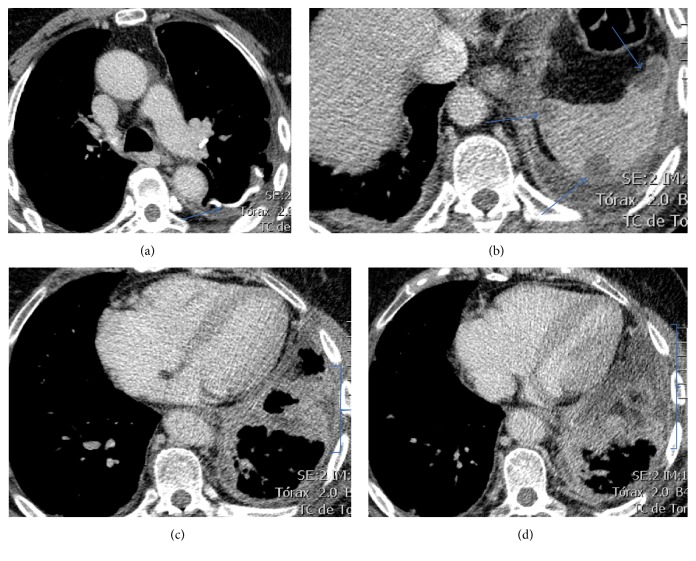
Chest CT. Multislice chest axial CT-images on mediastinal window show (a) signs of previous atypical wedge resection in the left lower lobe (arrow); (b) more nodular lesions in the spleen (arrows); (c and d) more pronounced and diffuse pleural invasion (brackets).
